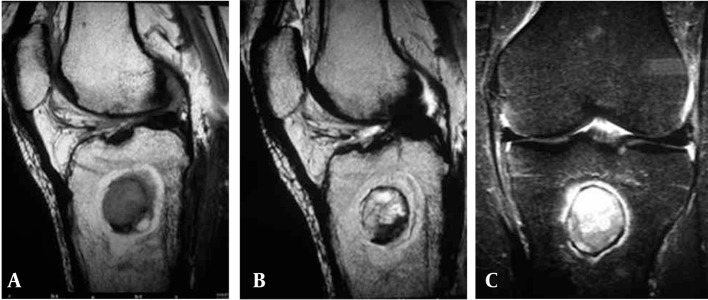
MRI of the same patient; A, T1-weighted TSE sequence reveals the penumbra sign; B, T2-weighted TSE sequence reveals the penumbra sign; C, MR image STIR-TSE sequence shows the penumbra sign.
